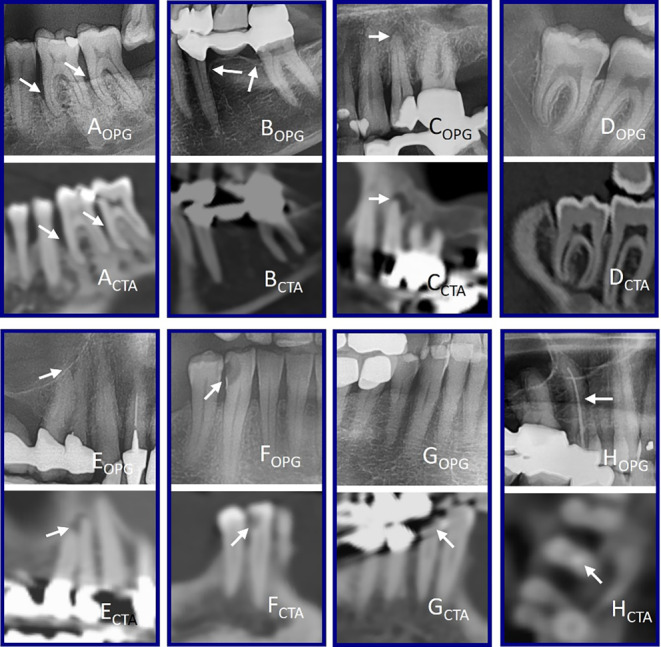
Exemplary dental findings in CTA and OPG. Exemplary dental findings in OPGs (upper image) and the respective CTA (lower image). a Arrows mark periodontal intra-bony defects, identified by all raters both in CTA and OPG. b OPG reveals periodontal bone defects (identified by all raters) that were not identified in CTA due to beam-hardening artefacts. c Arrow marks a periapical radiolucency, that was identified by all raters in both modalities. d Third molar identified as partially retained by two raters in OPG and by all raters in CTA. e Arrow indicates a periapical radiolucency, identified by 2/3 raters in OPG and by all raters in CTA. f Arrows mark carious lesions, which were identified by all raters in both modalities. g Arrow marks beam hardening artefacts that were misinterpreted as carious lesions by one rater. h Arrow marks RCF identified in OPG by all raters and unidentified by one rater in CTA
